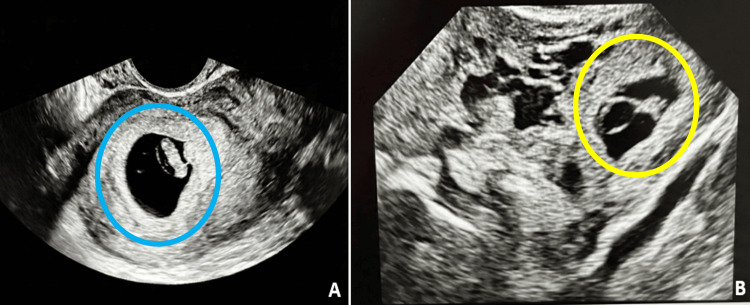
Obstetric ultrasound at 7 + 4 weeks of pregnancy. A. The blue circle shows a yolk sac at the intrauterine level. B. The yellow circle demonstrates the yolk sac in the right adnexa measuring 24 x 18 mm.
